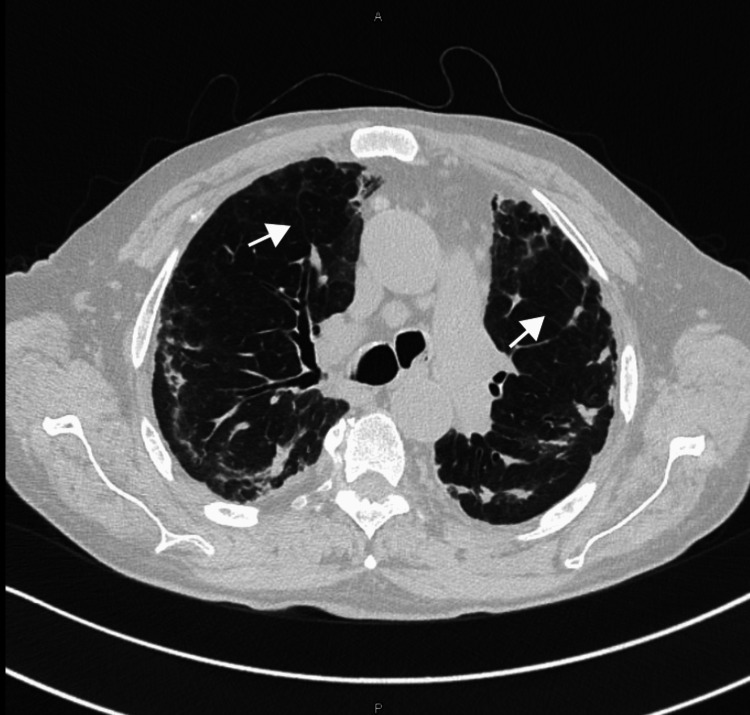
HRCT findings in chronic eosinophilic pneumonia High-resolution computed tomography (HRCT) of the chest showing diffuse mosaic attenuation patterns.
